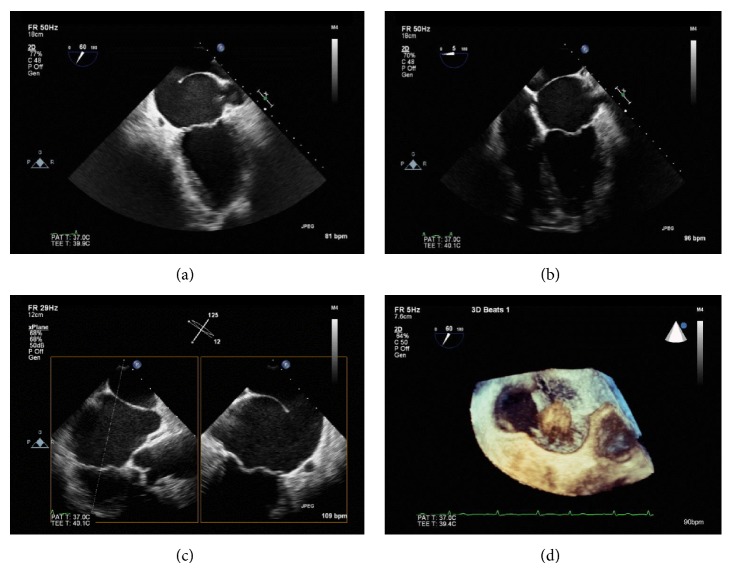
Transesophageal echocardiogram. 2-chamber view (a), 4 chamber view (b), biplane view (c), and 3D mode (d) showing the left atrium divided by a large fenestrated fibromuscular membrane consistent with cor triatriatum sinister.
